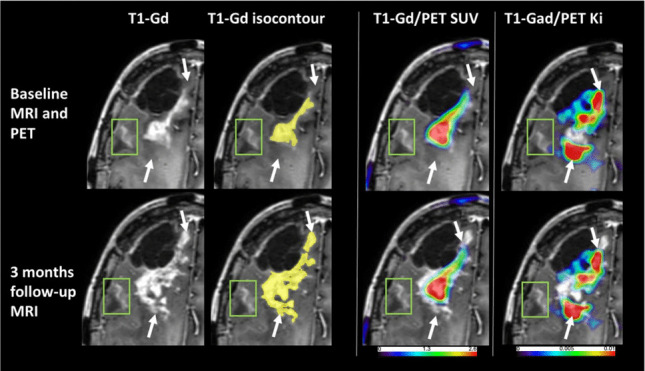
Detection of non-enhancing glioblastoma progression by post-treatment [^18^F]FETrp PET (patient #2). PET was done to evaluate two new contrast-enhancing lesions in the right frontal lobe > 6 months after resection and chemo-radiation. The right lateral frontal contrast-enhancing area (green box) showed no [^18^F]FETrp increases (both SUV and K_i_ below threshold); this area remained stable on subsequent MRI 3 months later, indicating radiation injury (bottom panel). The larger medial mass showed high [^18^F]FETrp SUV mostly confined within the contrast-enhancing volume, while high K_i_-values extended behind (also anterior to) this mass into non-enhancing brain (arrows). Follow-up MRI 3 months later showed expansion of contrast enhancement into these high-K_i_ areas, consistent with tumor progression in this lesion. Corresponding time-activity curves of this patient representing regions with high Ki and high SUV values together with Patlak-plots are provided in the Supplementary Data section
